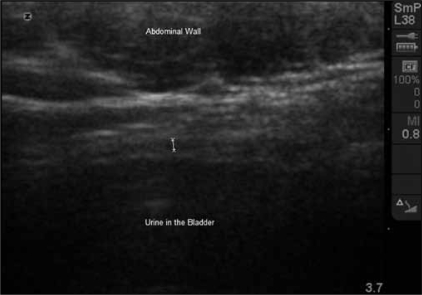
Trans-abdominal ultrasound image of the bladder at 3.7 MHz showing the abdominal wall and bladder. The detrusor is identified as a hypoechoic region sandwiched between an inner and outer hyperechoic layer characteristic of the mucosa and adventitia, respectively
